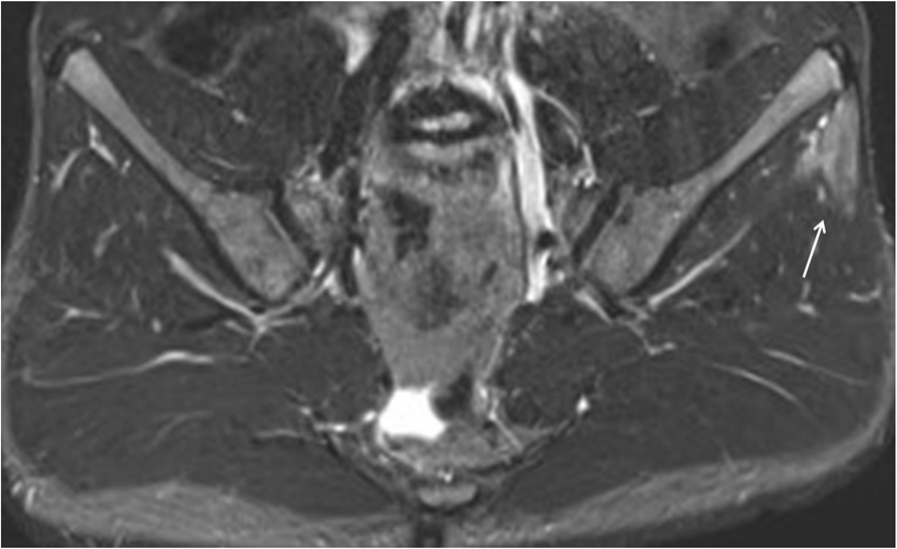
Enthesitis of the left gluteus medius origin in a 15-year-old boy with arthralgias (ERA-negative according to ILAR). Semicoronal STIR MR image of the SI-joint shows edema at the origin of the gluteus medius muscle at the iliac crest representing enthesitis
